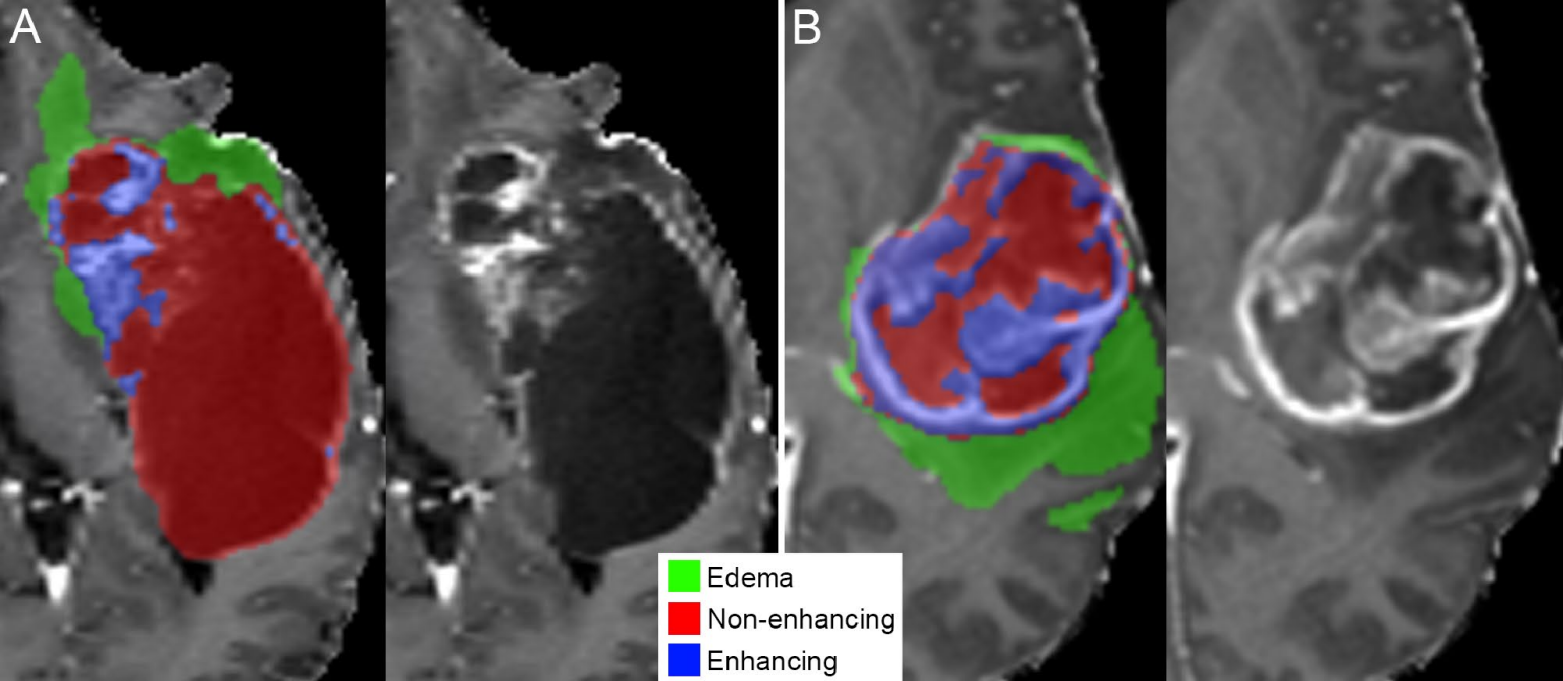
Representative example of tumor subcompartment segmentations for two glioblastomas, *IDH* mutant (A) and chromosome 7/10 aneuploid (B). Tumor subcompartment color overlays (see legend) are shown on top of postcontrast T1-weighted images (left of each panel) and compared with the same postcontrast T1-weighted image slice without a color overlay (right of each panel).
